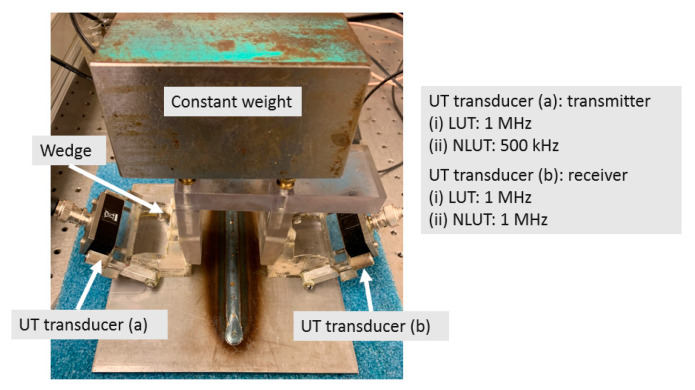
Experimental setups of LUT (Linear Ultrasonic Testing) and NLUT (Nonlinear Ultrasonic Testing).
